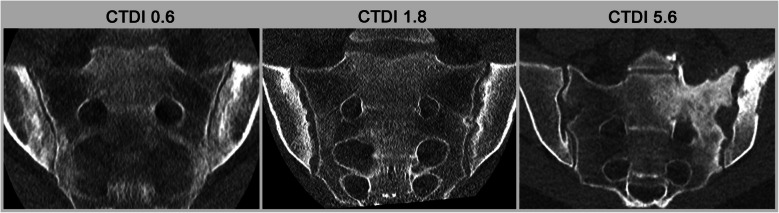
Comparison of ultra-low-dose (ULD)-CT, low-dose (LD-)CT, and standard CT for sacroiliitis. The three patients present with comparable age and weight. Images for ULD-CT and LD-CT were reconstructed with artificial intelligence, while the standard CT used iterative reconstructions. In all patients, structural lesions compatible with sacroiliitis are clearly visualized. While ULD-CT utilizes only 10% radiation of the standard CT, it also shows some degradation in image quality with loss of clear delineation of the cortical bone. CTDI is presented as mGy
